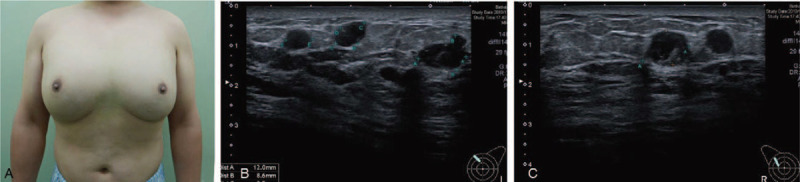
Preoperative picture and sonography examinations. A. Preoperative anterior view. B. Preoperative left breast sonography. C. Preoperative right breast sonography.
